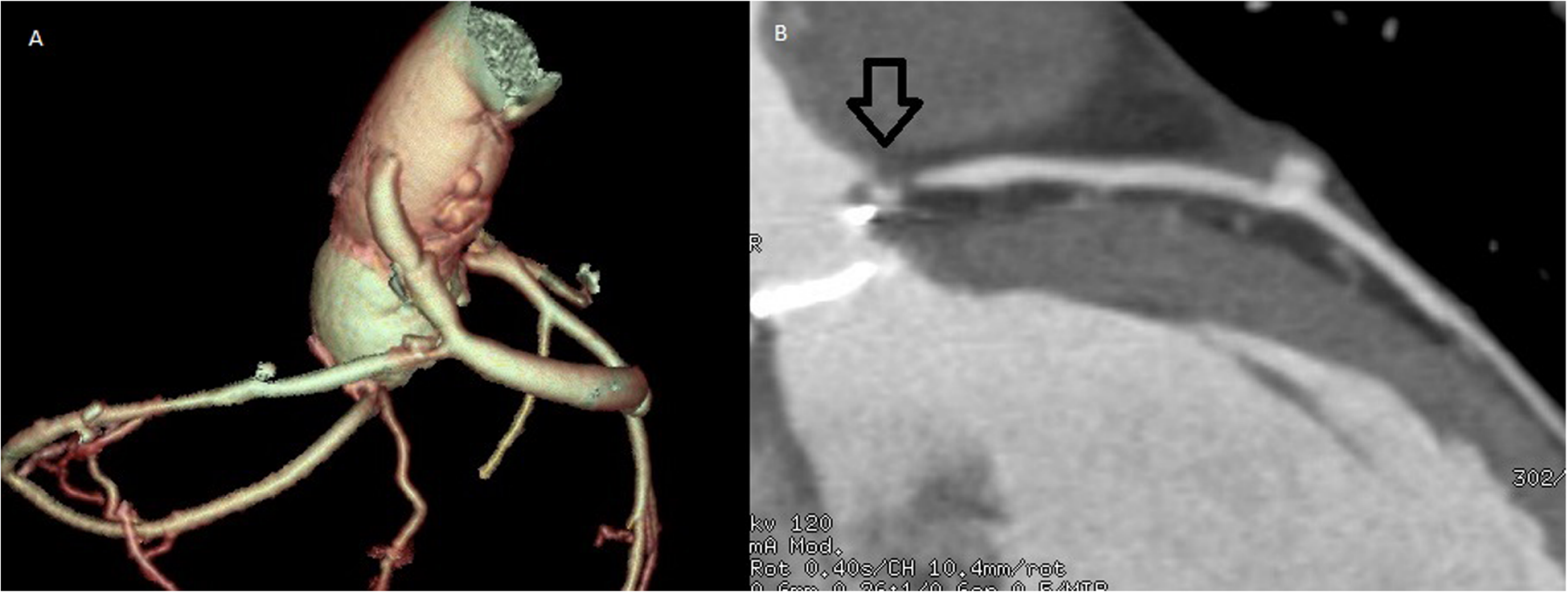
a CT-coronary angiogram revealing total ostial obstruction of both coronary arteries. b CT-coronary angiogram revealing obstruction on the left coronary artery
